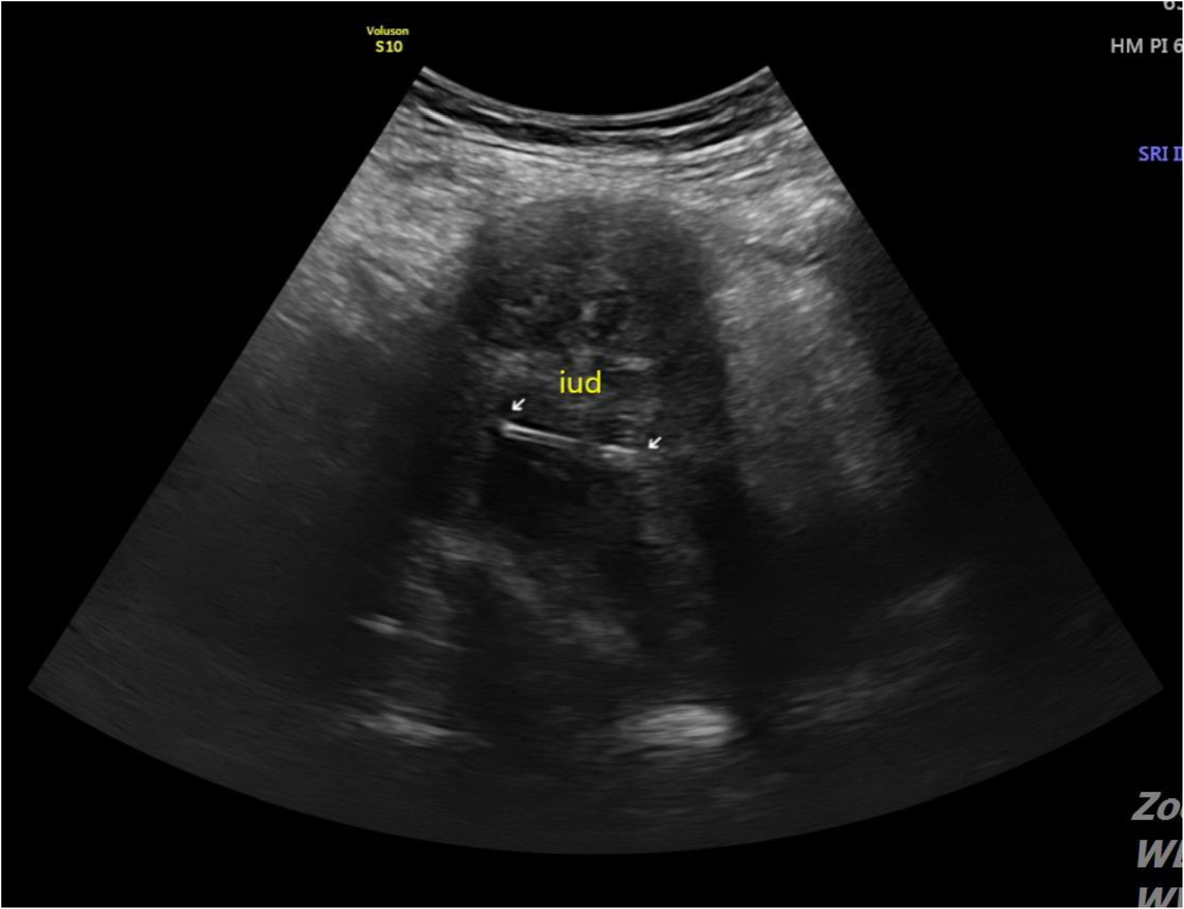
Ultrasound revealed an intra-uterine device (IUD)
